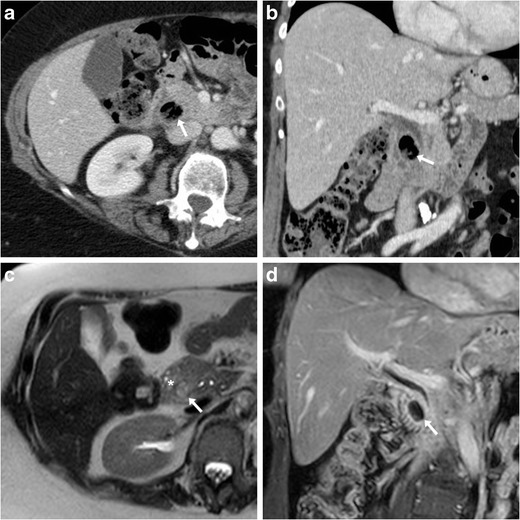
In a 77-year-old female with recurrent upper abdominal pain, axial (a) and coronal (b) post-contrast CT images showed a duodenal diverticulum (arrows) filled by air and enteral material, in typical medial site at distal descending duodenum. At MRI, T2-weighted (c) and fat-suppressed gadolinium-enhanced T1-weighted (d) images respectively showed oedematous, inflamed surrounding pancreatic parenchyma (+) and hyperenhancing contour of diverticulum (arrows)
